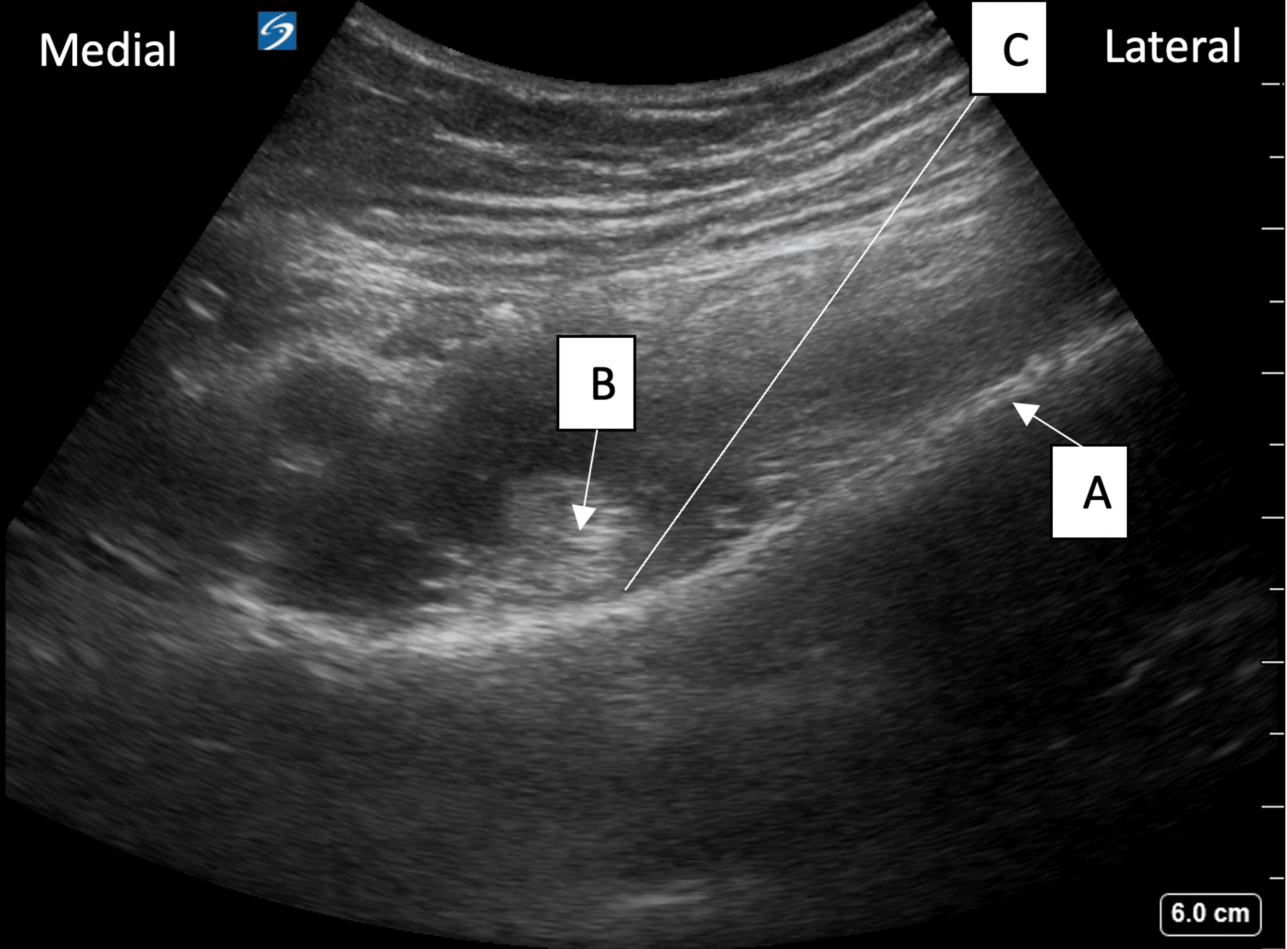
An example of PENG ultrasound anatomy The image shows the anterior inferior iliac spine (A), psoas tendon (B), and trajectory of the needle (C). PENG: Pericapsular nerve group.
